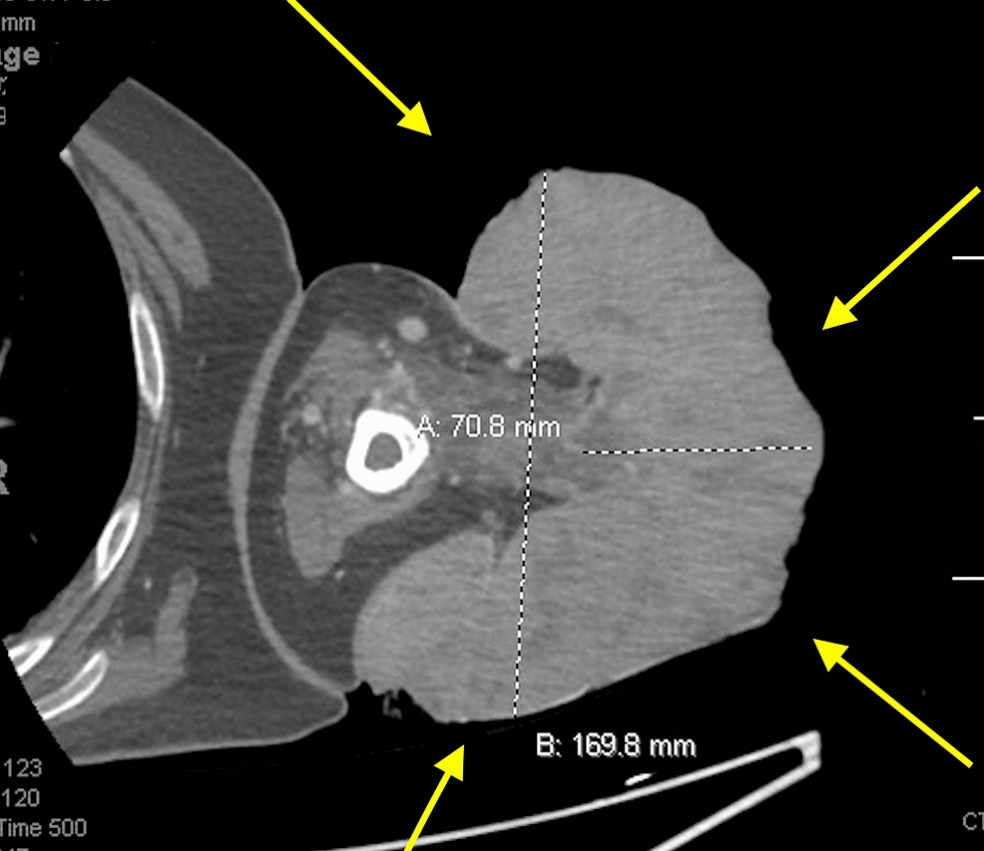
Upper extremity CT with contrast, unveiling a substantial pedunculated fungating mass, measuring approximately 17 x 14 x 8 cm, originating from the proximal lateral arm.
